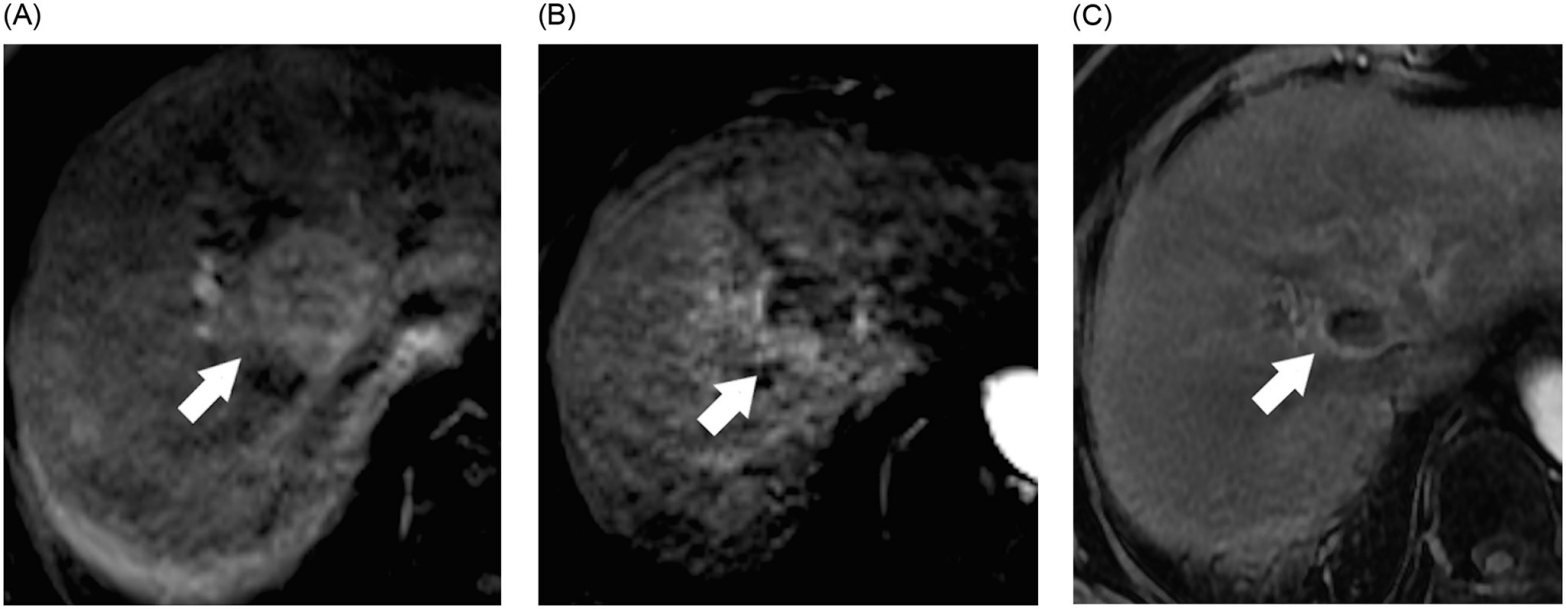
Early tumor regression (ETR) of the hepatocellular carcinoma (HCC) after proton beam therapy (PBT). 74-year-old woman with cirrhosis from chronic hepatitis C virus infection and HCC. MR image obtained before (A), 1 month (B) and 5 months after (C) PBT are shown. (A) Axial T1-weighted image during arterial phase obtained before PBT shows a 3.7 cm HCC in segment 8 (arrow). (B) Axial T1-weighted image during the arterial phase obtained 1 month after PBT shows ETR of the tumor with greater than 50% decrease of diameter of viable tumor (arrow) compared with pretreatment image. (C) Axial T1-weighted image during the arterial phase obtained 5 months after PBT shows lack of enhancement in lesion (arrow); this finding is compatible with complete response.
